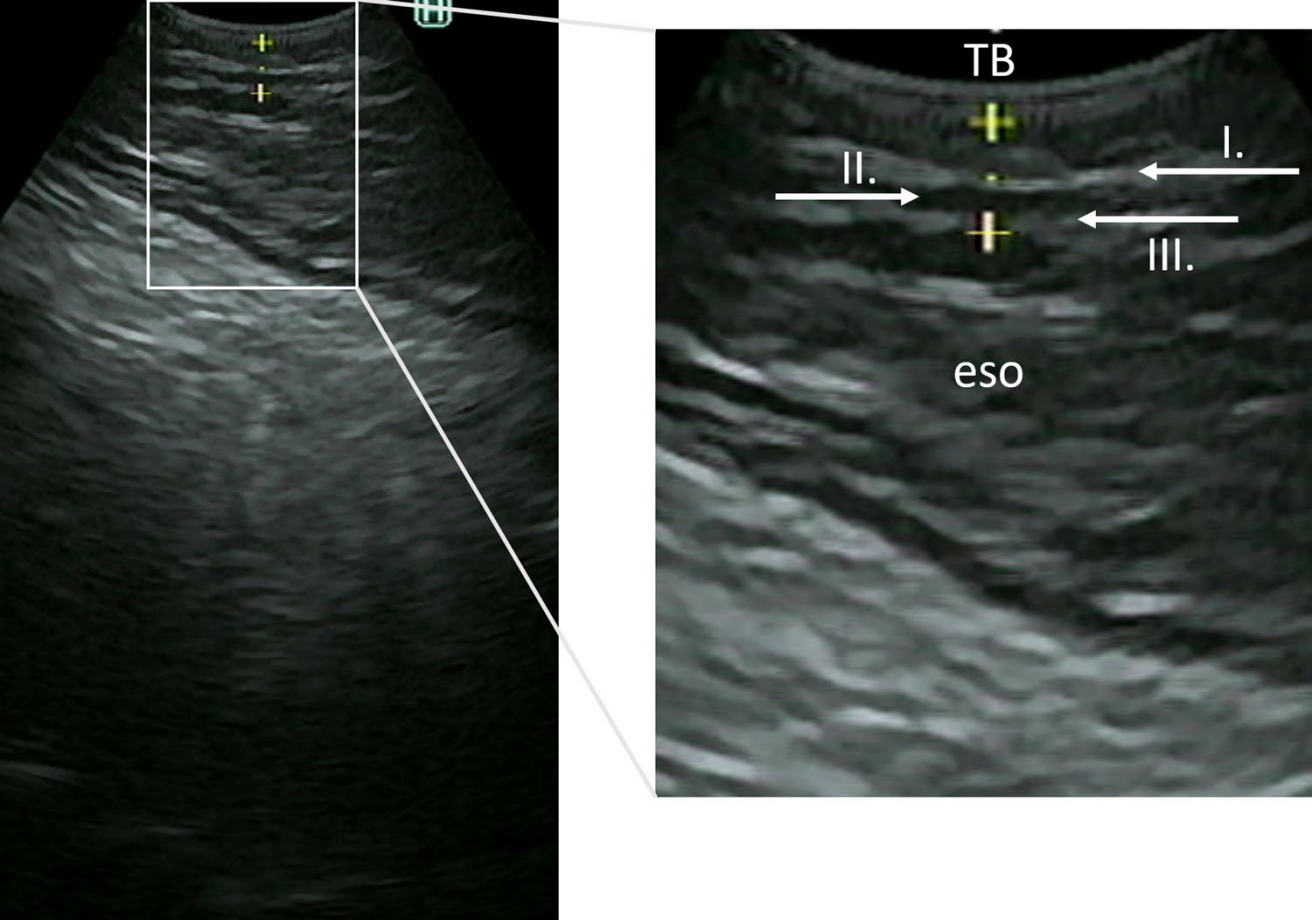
An EBUS image of the tracheobronchial wall as seen in the absence of tumor invasion. Three distinct layers (hyperechogenic, hypoechogenic, and hyperechogenic) are shown. The third layer corresponds with the adventitia of the membranous part of the tracheal wall. EBUS, endobronchial ultrasonography; eso, esophagus; TB, tracheobronchial tree
